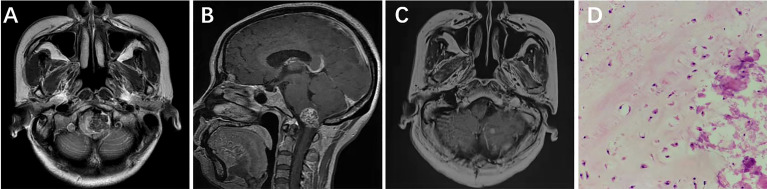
Left side of the medulla oblongata showed a round mixed short T2 signal shadow within which speckled long T2 signal was seen (A). Sagittal contrast-enhanced MRI showed that medulla oblongata was compressed by confounding signal mass located in foramen magnum (B). Six months after surgery, enhanced MRI showed that the tumor had not recurred (C). Pathological results at 200× magnification showed mature chondrocytes of different sizes and vacuolar formation in some cells (D).
